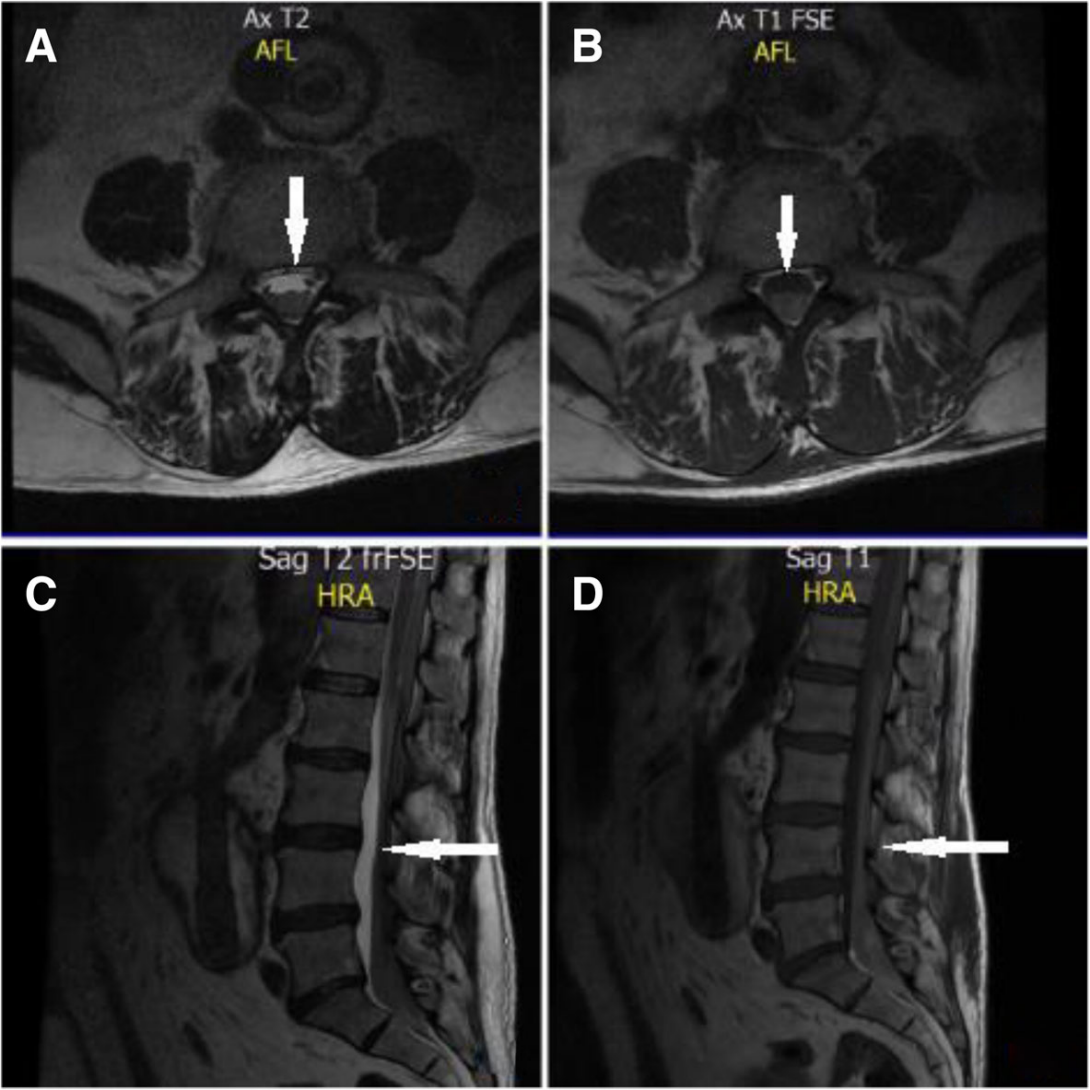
a Magnetic resonance imaging (MRI) T2-weighted transverse section. A hyperintensity zone is observed showing spinal infarction at the T10 level. b MRI T1-weighted hypointensity showing spinal cord infarction. c MRI T2-weighted sagittal section showing extension of the medullary infarction with hyperintensity zone. d MRI T1-weighted image showing extension of the medullary infarction with hypointensity zone. The area of infarction is indicated by the *white arrow*
